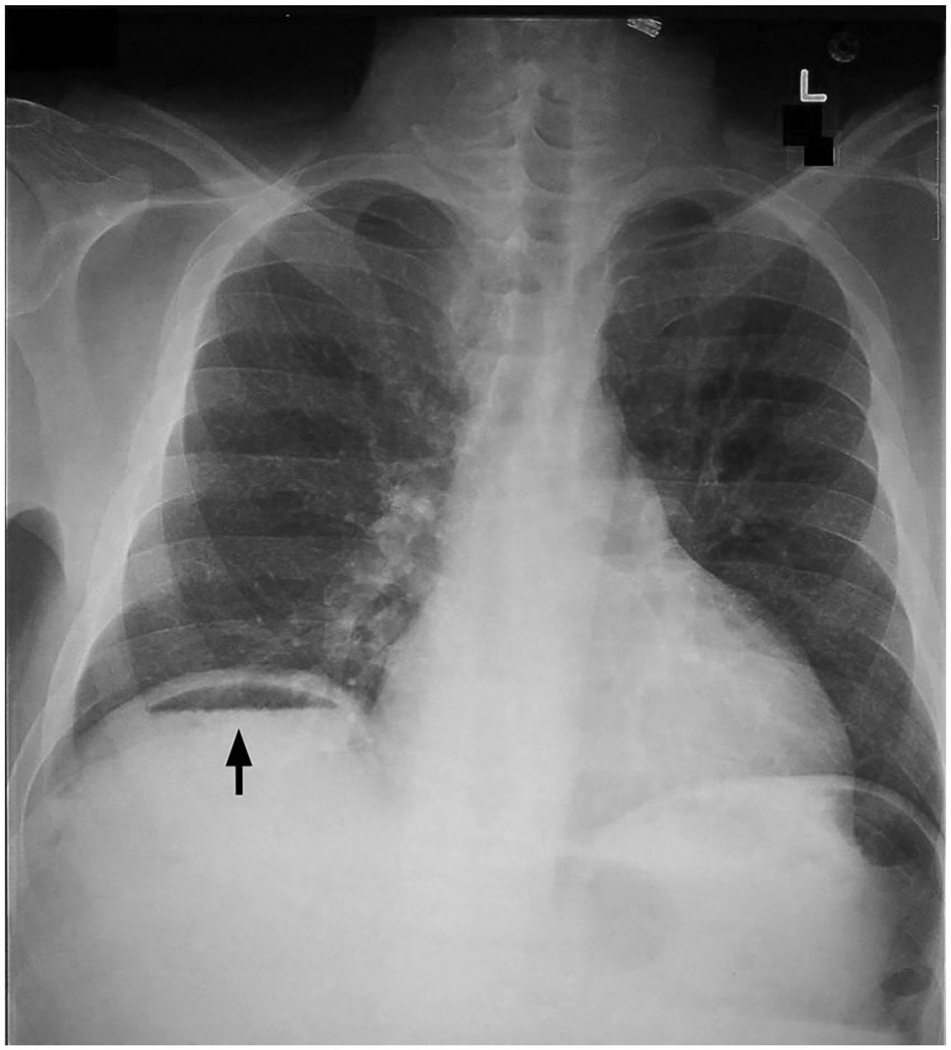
Chest x-ray of the patient shows the presence of air located under the diaphragm.
